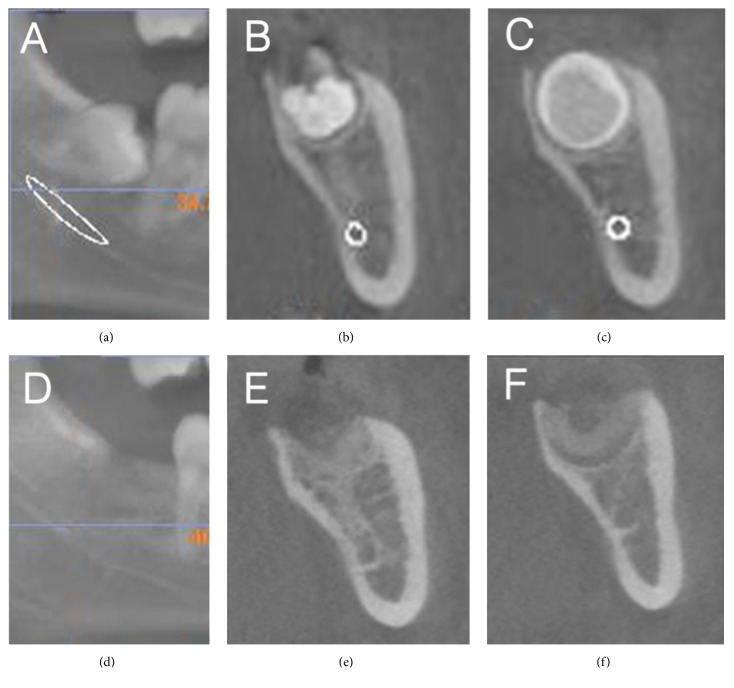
CBCT before (a, b, c) and 3 months after (d, e, f) M3M removal and socket preservation with beta-TCP bone filling material. Sagittal representation (a, d) and cross-sectional reformats at 3 and 6 mm distance to the second molar (b, e) and (c, f). The cross sections (c) and (f) represent the center of the socket and were used for bone height measurements.
